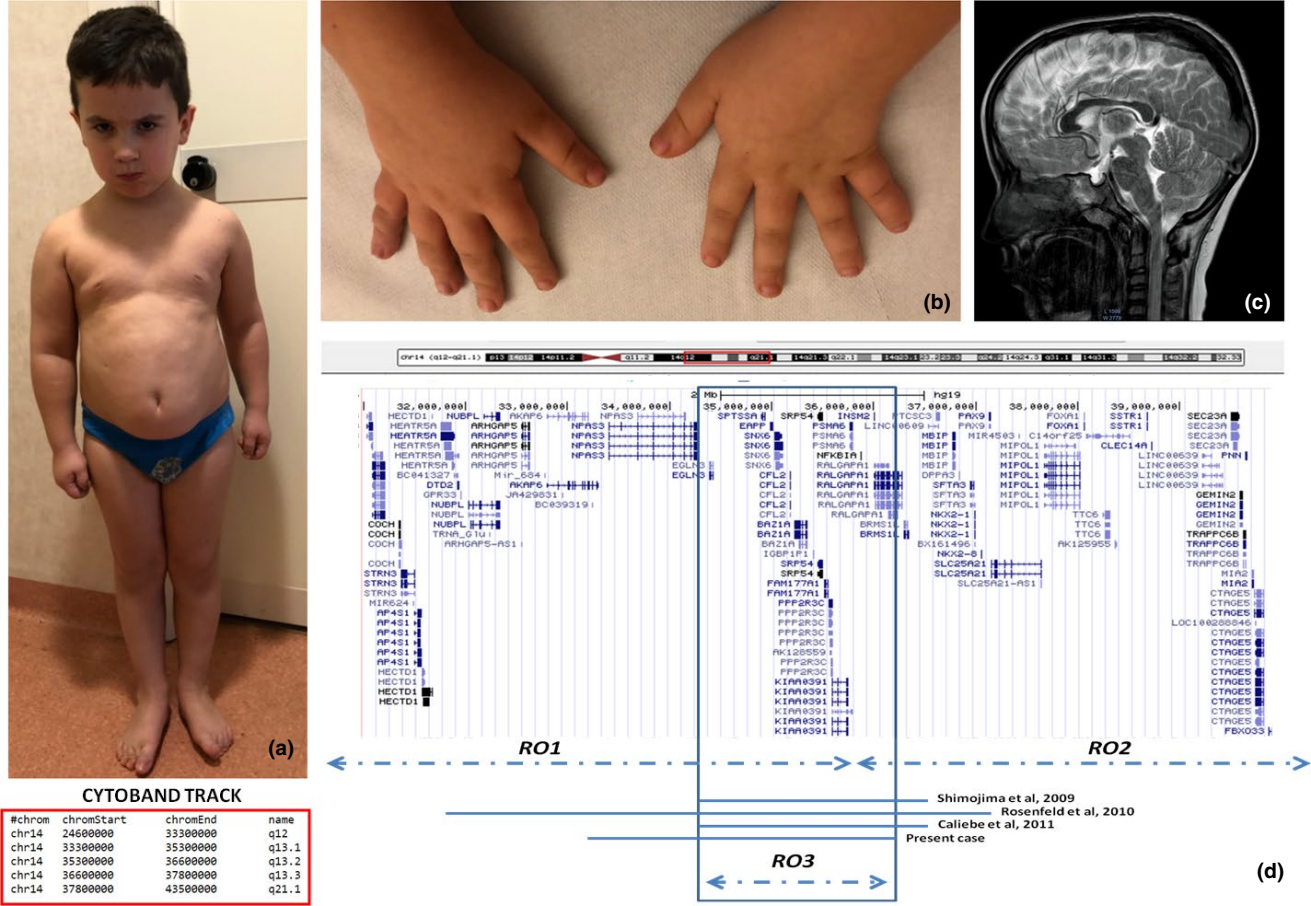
A‒C, Clinical photographs of index case at 6 years of age. Frontal view showing regional abdominal fat distribution (BMI > 99 centile), short neck, and facial features (A); Characteristic hands with stubby fingers (B); Brain magnetic resonance imaging (MRI) sagittal T2 image showing corpus callosum hypoplasia (C). D, Overview of the 14q12q13 deleted region and cytoband track in our case compared with the other patients reported in literature: delineation of the third Region of Overlap (RO3); GeneBank reference sequence for OMIM gene involved in the deleted region for which this data is known: *NPAS3*(NG_013036.2), *CFL2*(NG_012740.1), *PSMA6*(NG_011703.2), *NFKBIA*(NG_007571.1), *RALGAPA1*(NG_051667.1)
